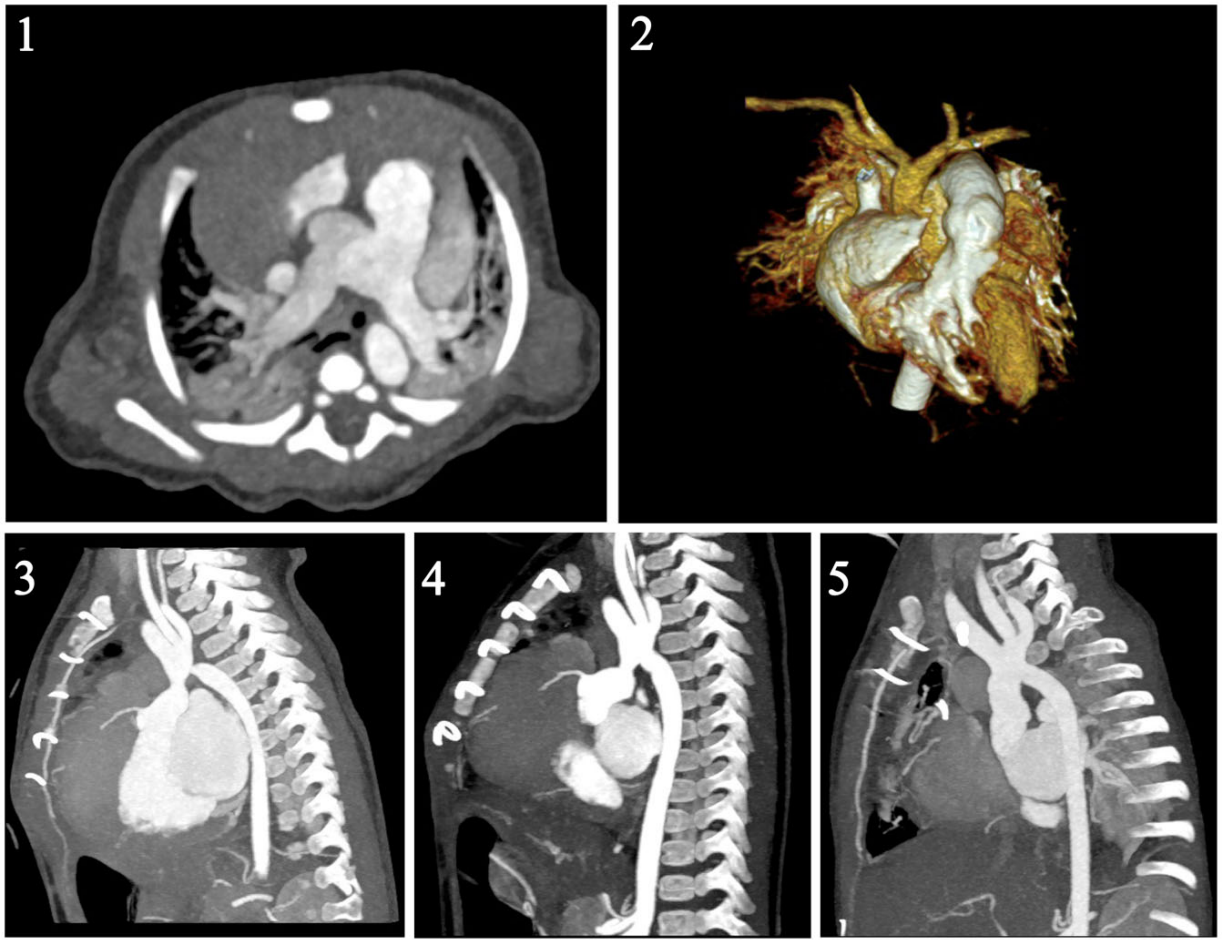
Images of ms-CT of preoperative APW-IAA and postoperative SAS: (1) APW type 3; (2) 3D-CT reconstruction of IAA type A and APW; (3) ms-CT angiogram shows SAS and aortic coarctation after single-stage repair; (4) ms-CT angiogram shows residual ascending aorta stenosis after reoperation; (5) ms-CT angiogram after the second reoperation shows no residual SAS or coarctation. APW: aortopulmonary window; IAA: interrupted aortic arch; ms-CT: multislice computed tomography; SAS: supravalvar aortic stenosis.
